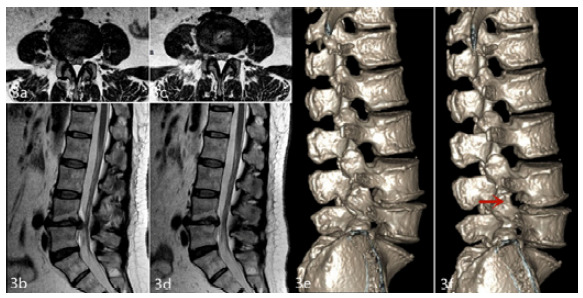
Representative lumber disc herniation case pre- and post-operation (L4-5, right paracentral type, female). Preoperative lumbar MRI showed that the L4-5 intervertebral disc was herniated to the right and that the L5 nerve root and dural sac were compressed (a, b); 12 months after the operation, lumbar MRI showed that the herniated intervertebral disc tissue had disappeared and that the right L5 nerve root and dural sac compression were relieved (c, d); Comparison of a three-dimensional CT reconstruction of the lumbar spine before and 12 months after the operation (the arrow indicates the L4-5 intervertebral foramen post-operation enlargement) (e, f).
